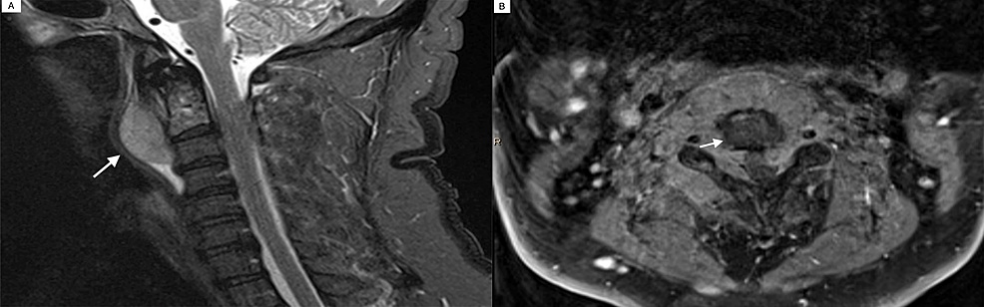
Magnetic resonance imaging (MRI) of the mass lesion A: sagittal T2 showed a retropharyngeal mass at the level of C2 extending to the C3 level. B: axial T1 postcontrast showed moderate to severe central canal narrowing.
